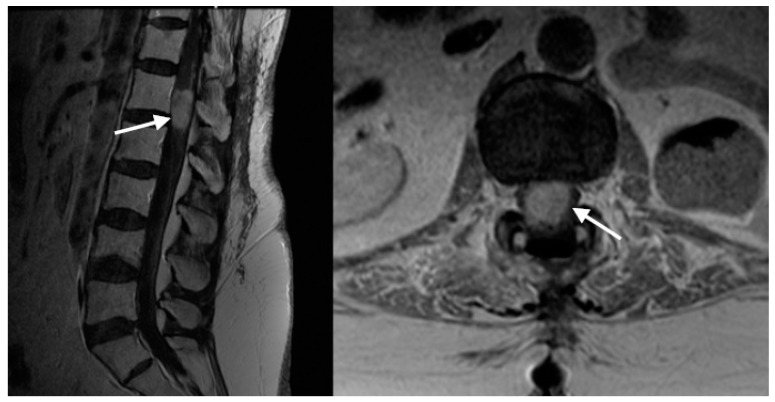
Sagittal and axial contrast-enhanced T1W MRI images show an enhancing lesion, compatible with conus medullaris metastasis (white arrows).
